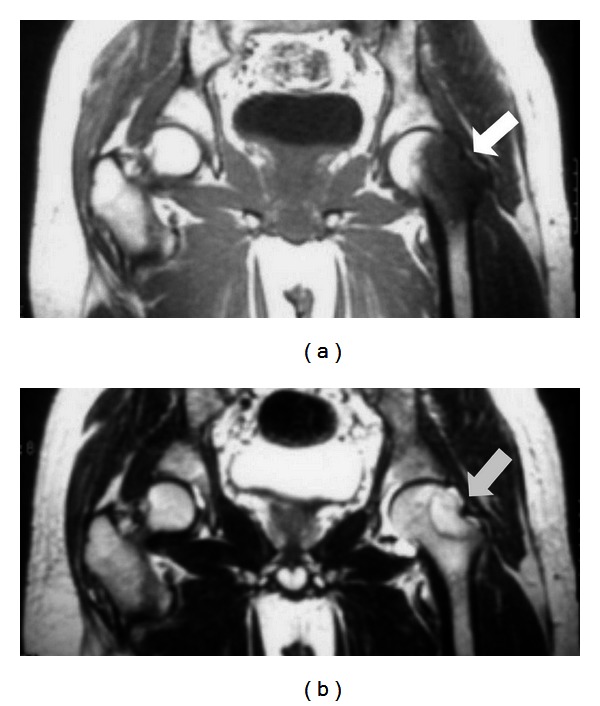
Coronal MRI pelvis showing (a) hypointense signal area in the marrow on T1-weighted image and (b) hyperintense signal area in the marrow on T2-weighted image, and there is no obvious extension of lesion into surrounding soft tissue.
